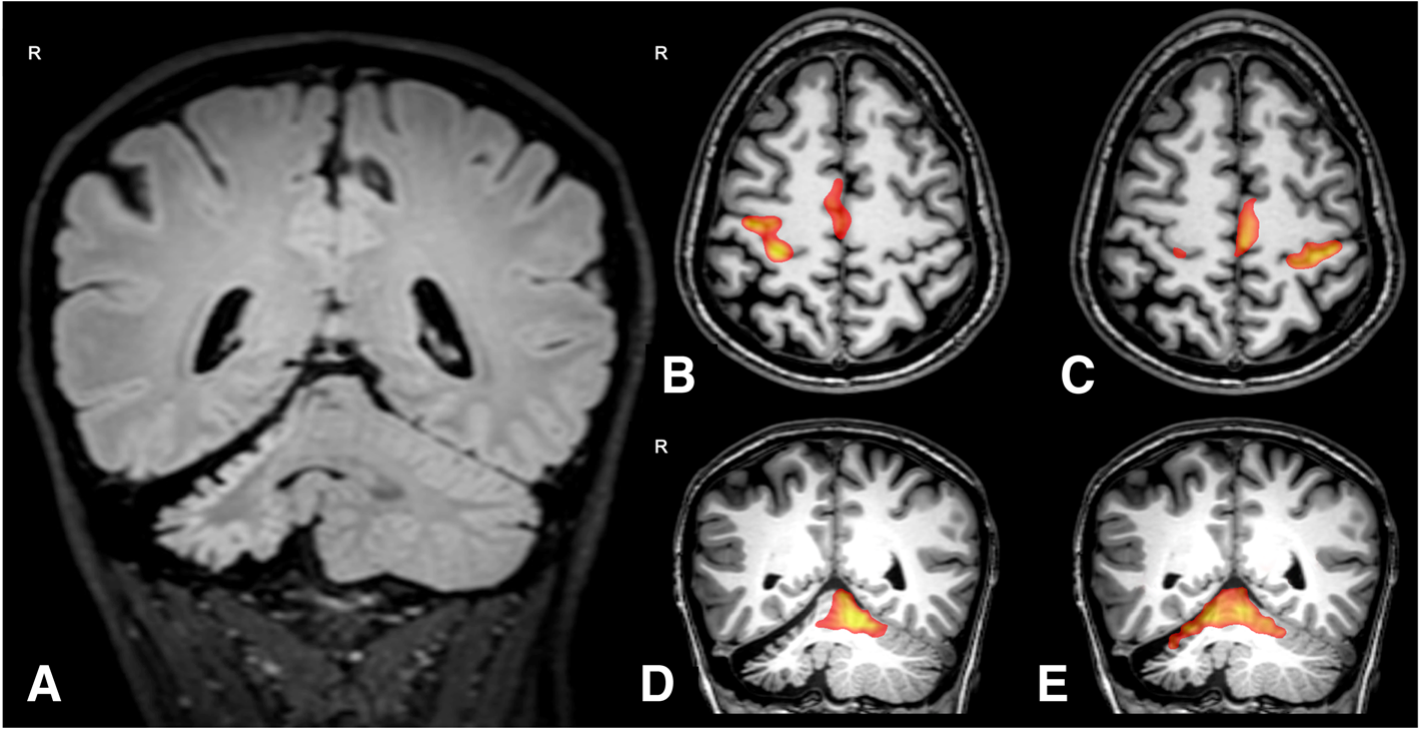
Coronal FLAIR image (a) shows markedly decreased volume of the right cerebellar hemisphere with hyperintensity of the right cerebellar cortex. Axial and coronal T1 weighted images with superimposed activated clusters during left (b and d) and right-hand (c and e) tapping. During left hand tapping the right precentral/postcentral gyri, right superior frontal gyrus and the left superior cerebellum are activated, whereas during right hand tapping the precentral/postcentral gyri on both sides, the left superior frontal gyrus and the bilateral superior cerebellum including the vermis are activated
